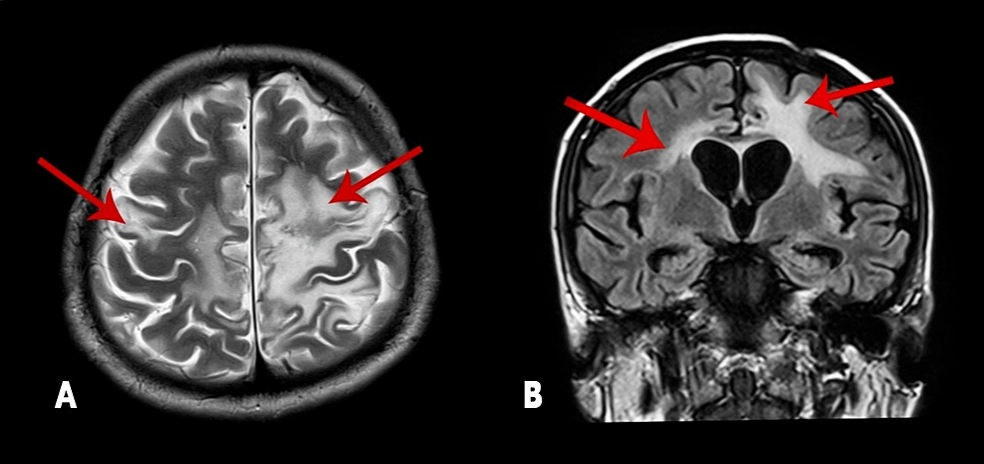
Magnetic resonance imaging of the brain after chemotherapy. A: Axial, T2 section showing significant disease reduction as seen by T2/FLAIR white matter signal abnormality within bilateral supratentorial cerebral hemispheres; B: Coronal view showing significant reduction in the disease process. FLAIR: Fluid-attenuated inversion recovery
